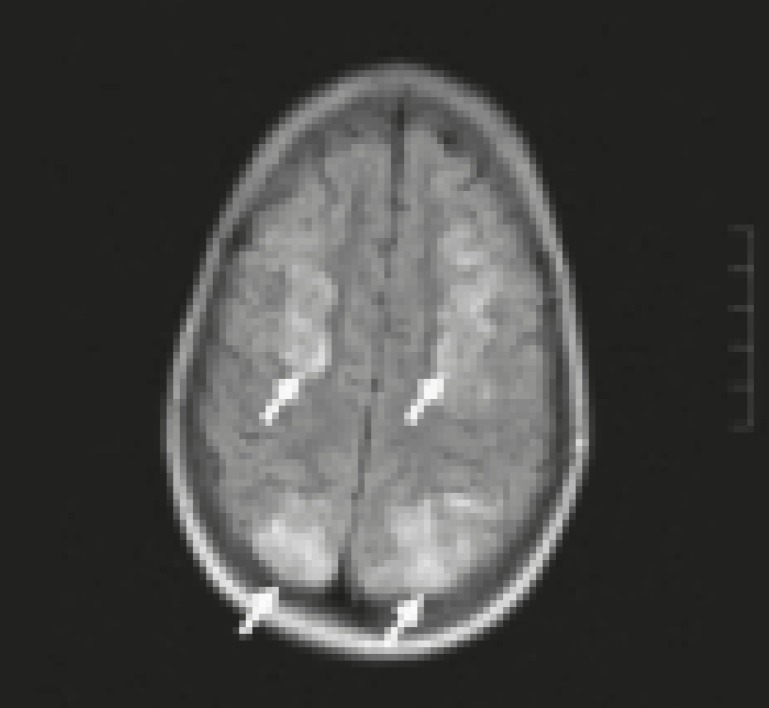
MRI in patient with PRES: Typical hyperintense spots at cortical-subcortical level in T2-weighted
